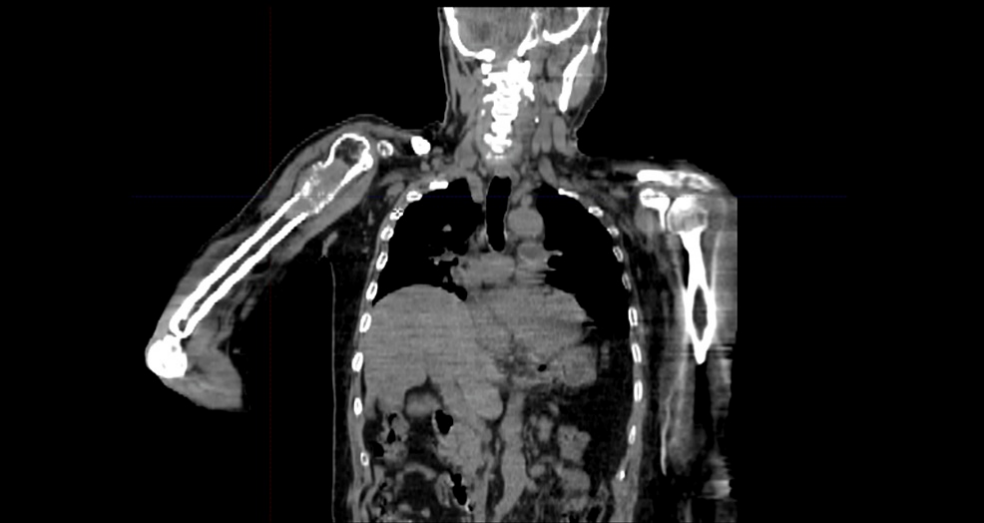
Computed tomography (CT) showing expansile lesion in the upper third of the right humeral bone.
